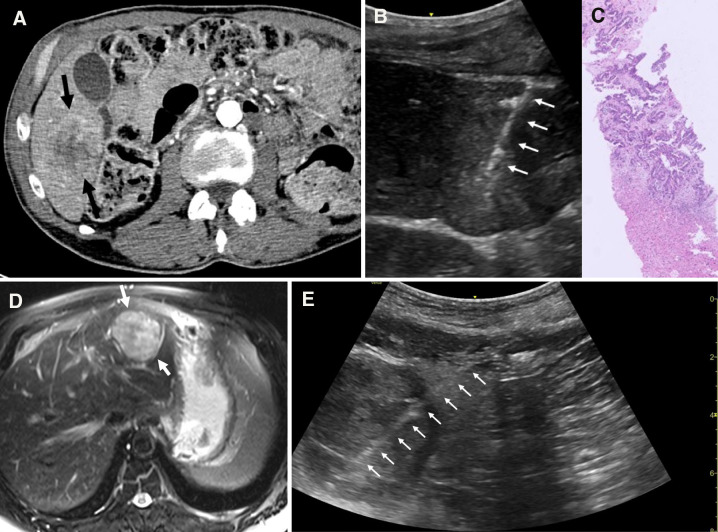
Cases #1 & 2, Panel (A) Case #1 liver mass seen by pre-biopsy CT imaging (black arrows). Panel (B) Case #1 cautery tract following biopsy (white arrows). Panel (C) Case #1 pathology reveals irregular, infiltrative glandular structures with hyperchromatic nuclei in keeping with adenocarcinoma of colorectal origin. These malignant units are encased within a desmoplastic stroma, demonstrating a clear fibrotic interface that compresses upon the adjacent benign hepatic parenchyma. Panel (D) Case #2 pre-procedure T2 fat-saturated axial MRI image shows liver mass (white arrows). Panel (E) Case #2 echogenic needle tract (white arrows) is demonstrated in ultrasound image post-biopsy and SinglePass device electrocautery (white arrows).
